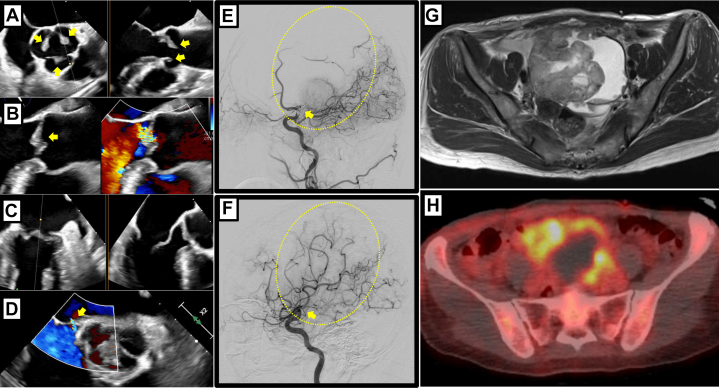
Imaging Before Tumor Resection (A and B) Mobile vegetations on all of the aortic valves (arrows) and mild aortic regurgitation (arrow) by TEE; the largest vegetation measured 7 × 6 mm on the left coronary cusp. (C) No vegetations were detected at the mitral valve. (D) A small atrial septal defect was found (arrow). (E and F) Occlusion and recanalization of the left middle cerebral artery demonstrated on sagittal view before and after the procedure (arrows). (G) MRI showing a solid mass with a maximum diameter of 9.2 cm, with contrast enhancement, diffusion restriction, and expansile features in the pelvic cavity. The origin of the lesion is presumed to be the left ovary. The cystic component showed mildly high signal intensity on T1-weighted imaging. (H) PET/CT demonstrated an irregular cystic mass compressing the uterus to the right side in the midline of the pelvis. Within the lesion, an area of thickened solid wall demonstrated intense and heterogeneous uptake of fluorine-18-fluorodeoxyglucose. There was no definitive evidence of distant metastasis. MRI = magnetic resonance imaging; PET/CT = positron emission tomography/computed tomography; TEE = transesophageal echocardiography.
